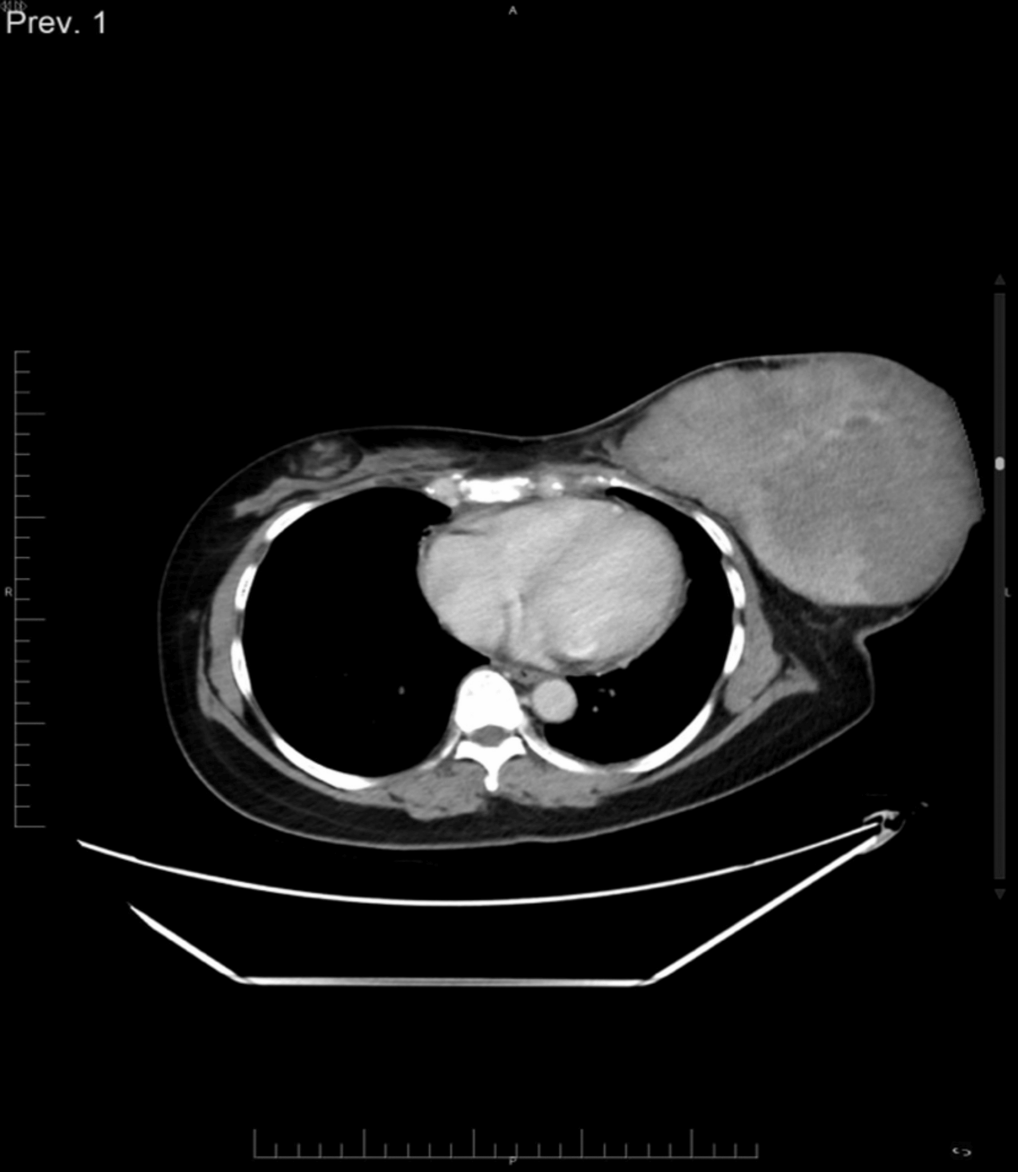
CT scan of the thorax showed large heterogeneously enhancing left breast fungating lesion involving most quadrants measuring around 17 × 12 cm
